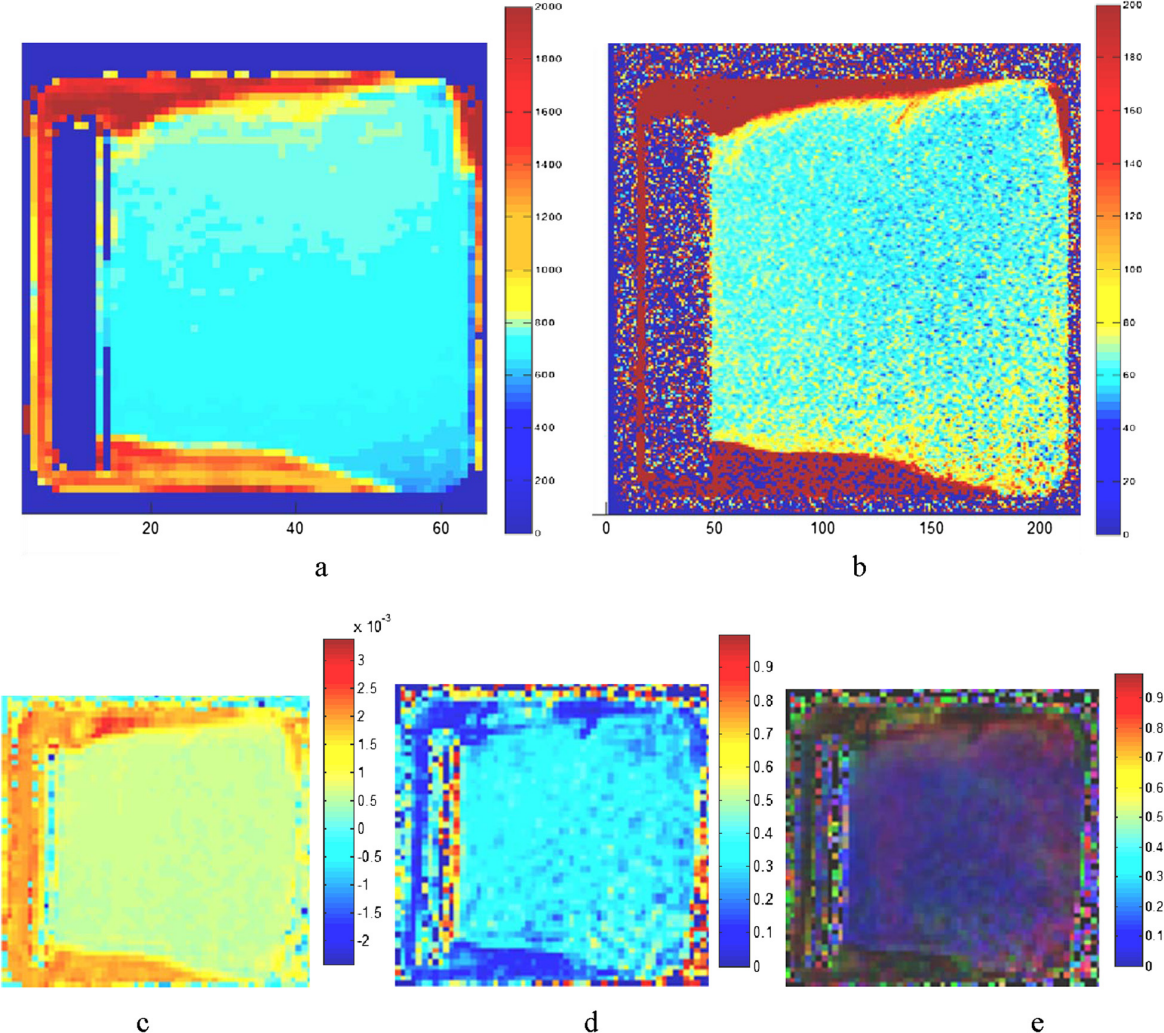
T1 in ms (a), T2 in ms (b), ADC in mm^2^/s (c), FA (d) and FA color (e) maps calculated from the T1- and T2-weighted images and from the diffusion tensor images of the sample shown in Figure1. For the FA color map, the colors indicate direction of fiber tracts (red transverse, blue cranio-caudal, green anterior–posterior).
